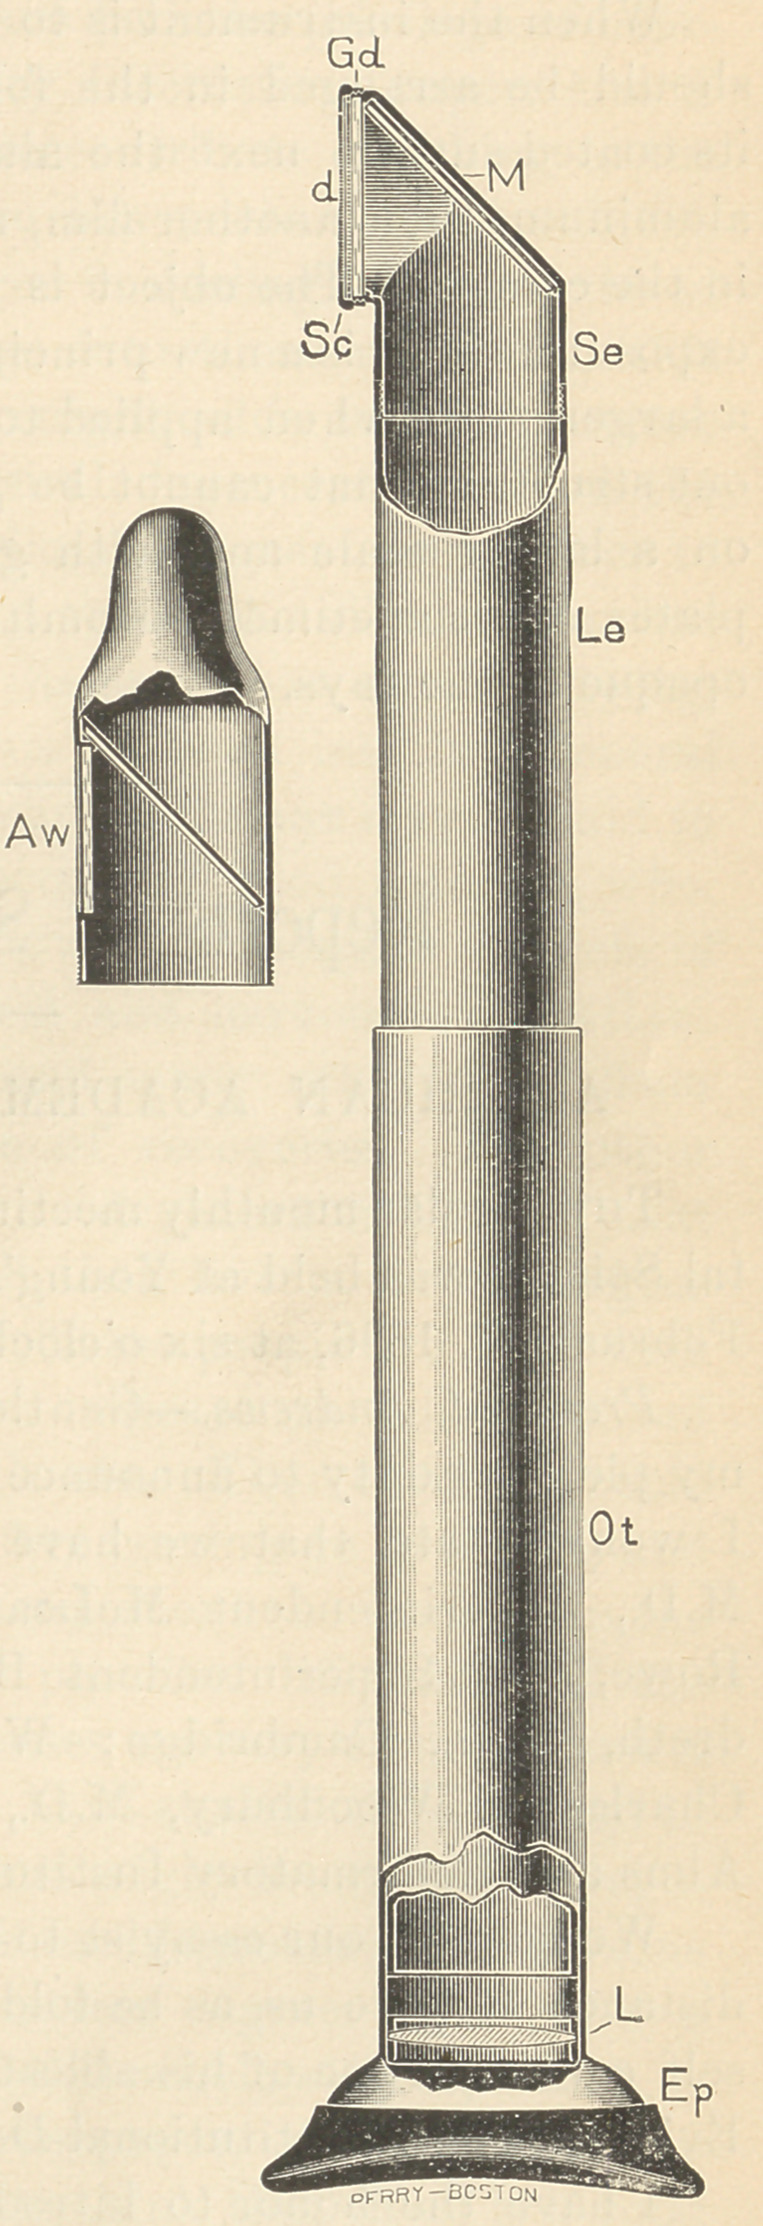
# A Roentgen Ray Converter for Internal Use
